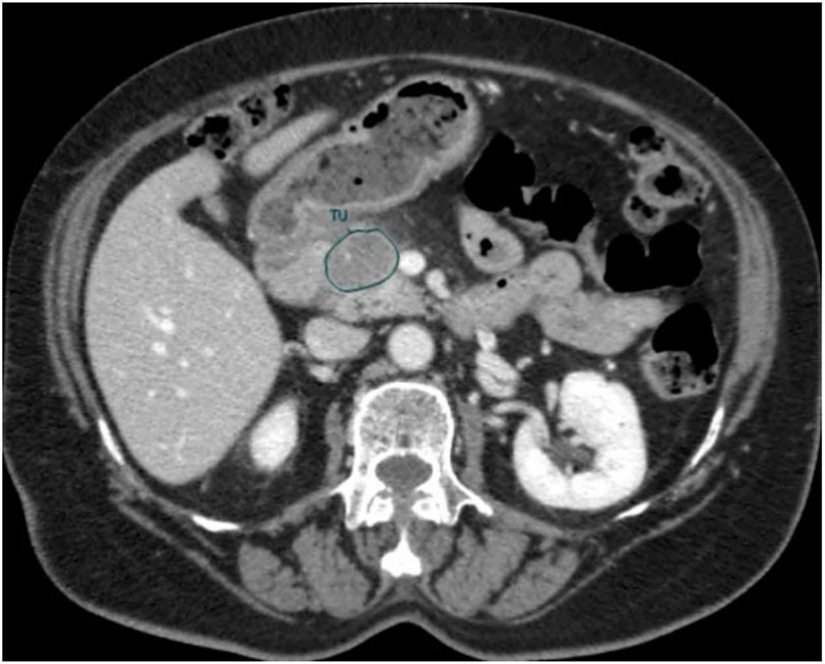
A manual contour of CT scan from a representative patient in cohort 2.
